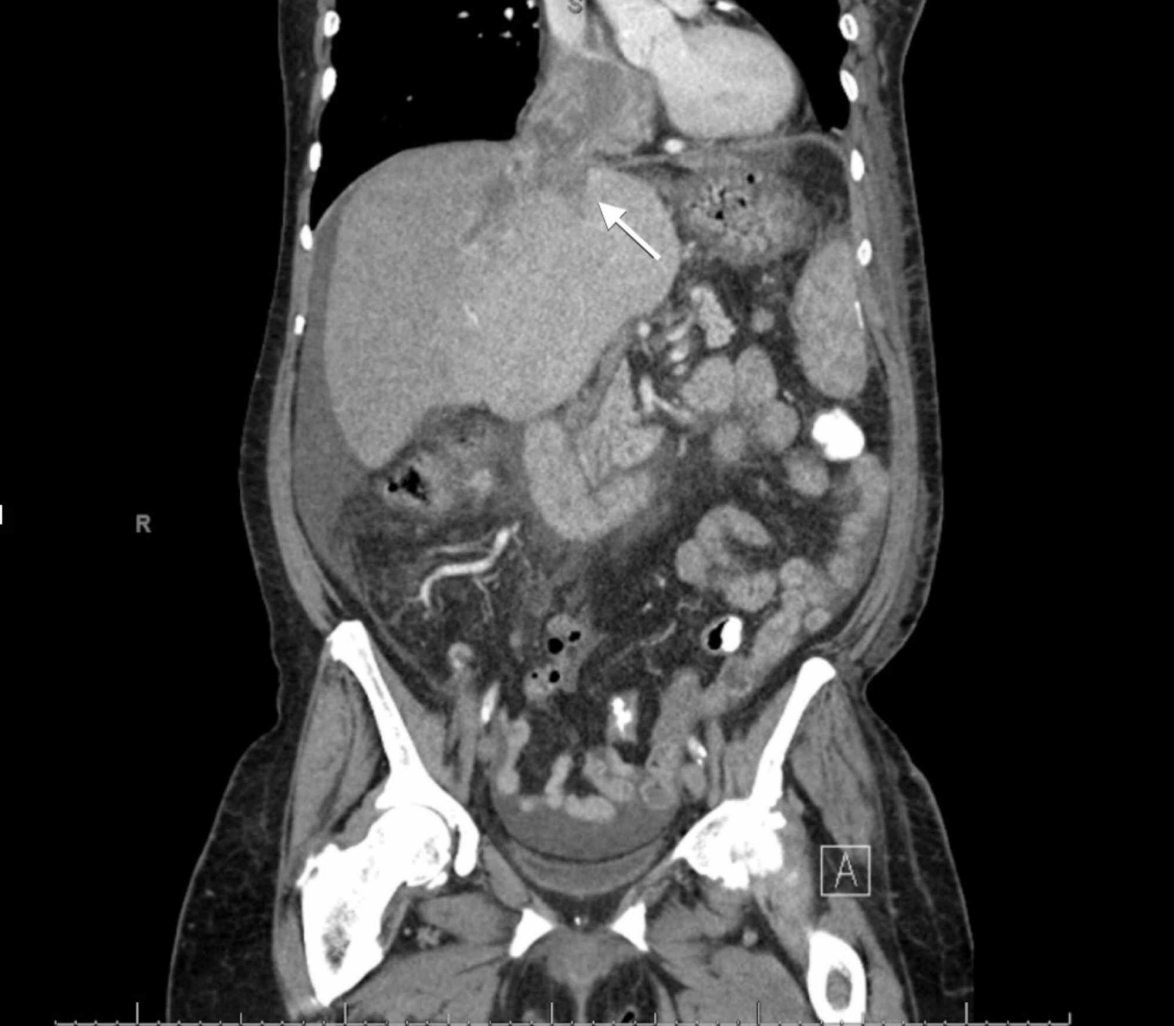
Triple-Phase Liver CT - Coronal view Coronal view of triple-phase liver CT scan revealed a tumor thrombus occupying the middle and left hepatic vein that partly extended into the right atrium.
